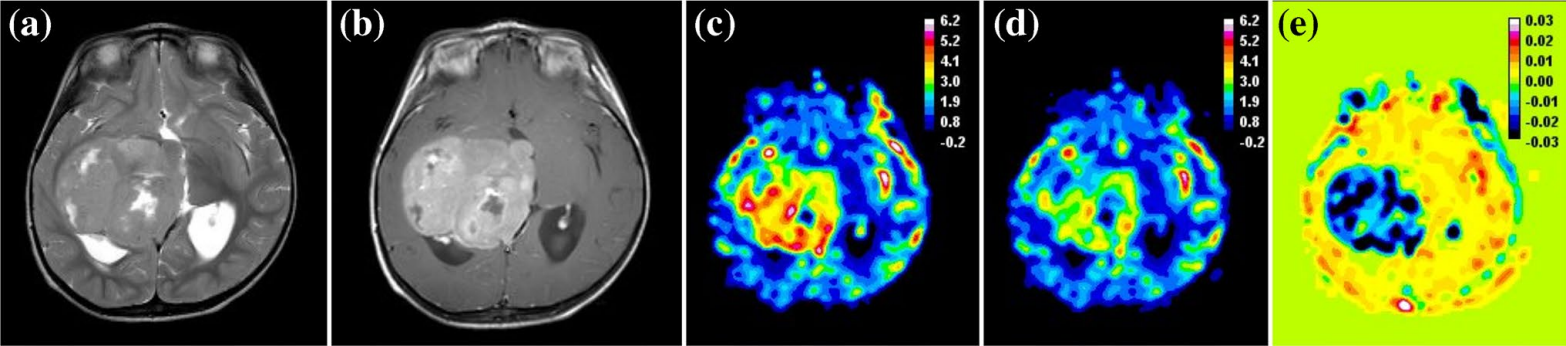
Images and maps from a high-grade tumor in a 2-year-old boy with a glioblastoma (classified as grade IV) scanned at center 4 on a 3-T Achieva (Philips, Best, the Netherlands). a Pre-contrast axial T2-weighted MR image (turbo spin-echo sequence, repetition time/echo time [TR/TE] = 6,272/85 ms, number of signals averaged [NSA] = 1, acquired with 4-mm slice thickness and 10% gap, reformatted to the dynamic susceptibility-contrast acquisition of 3.5-mm slice thickness, no gap and 1.8×1.8-mm in-plane resolution), on which the tumor region of interest was defined. b Post-contrast axial T1-weighted MR image (spin-echo sequence, TR/TE=1,179/14 ms, NSA=1, acquired with 4-mm slice thickness, 10% gap, reformatted as in (a) shows high signal in the tumor. c–e Uncorrected (c) and leakage-corrected (d) relative cerebral volume (rCBV) maps and K_2_ map (e) acquired in the axial plane. Dynamic susceptibility-contrast data were acquired with an sPRESTO sequence (TR/TE=15.9/23.9 ms, flip angle 7^o^, with 30 slices at 1.8×1.8×3.5-mm resolution). The uncorrected rCBV map shows high values within the tumor. After correction, rCBV is shown to decrease. The K_2_ map shows negative values within the tumor compared to surrounding normal tissue. Normalized uncorrected rCBV was high both before and after leakage correction (3.66 vs. 2.68) and K_2_ was −0.013. *sPRESTO* sensitivity-encoded Philips principles of echo-shifting with a train of observations
